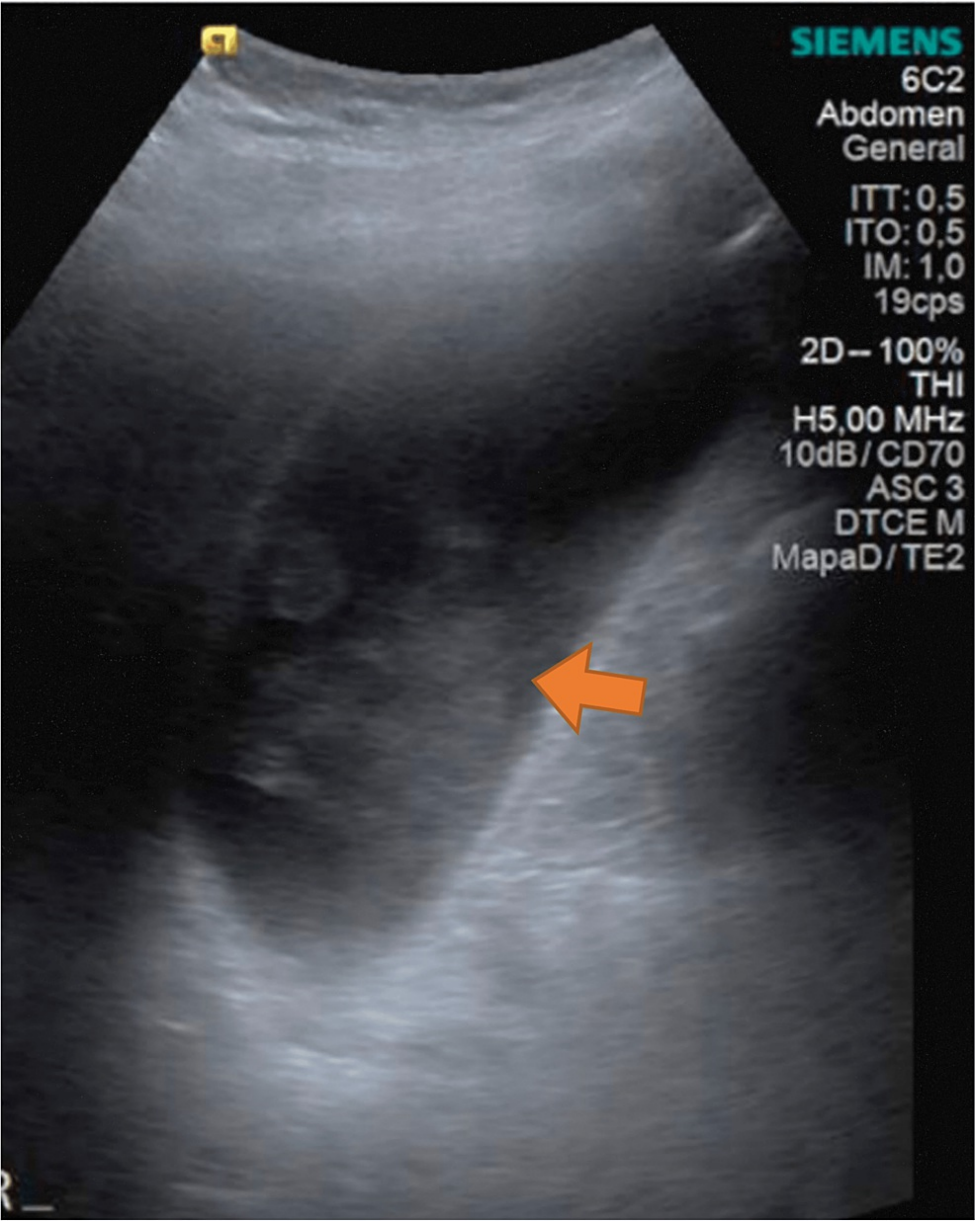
Gallbladder ultrasound showing a markedly dilated gallbladder with multiple small clustered images (orange arrow) suggesting biliary sludge
